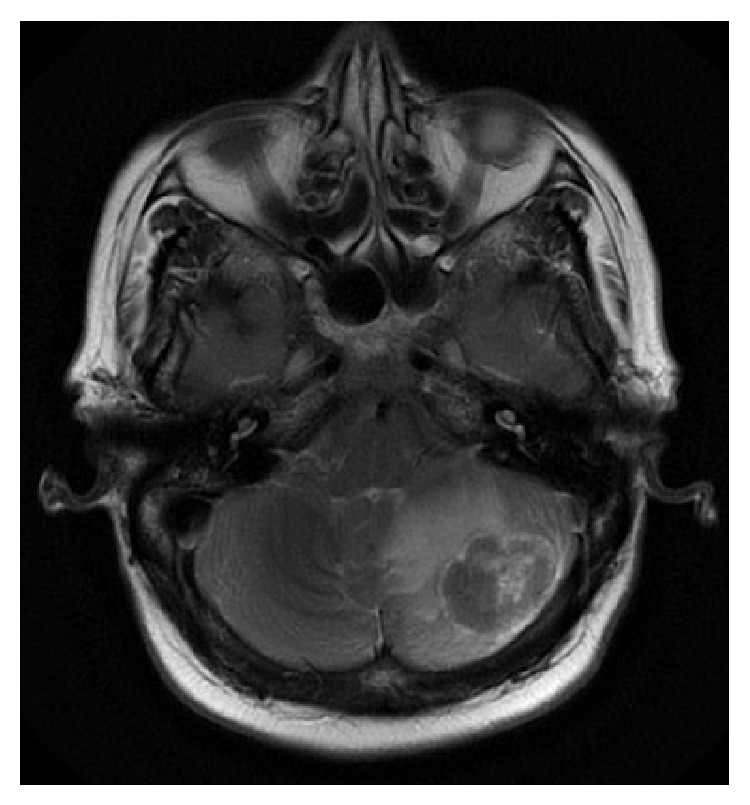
Magnetic resonance imaging (axial view) of brain showing a 4 × 4 cm heterogeneous mass in the left cerebellar hemisphere associated with edema (the third case).
